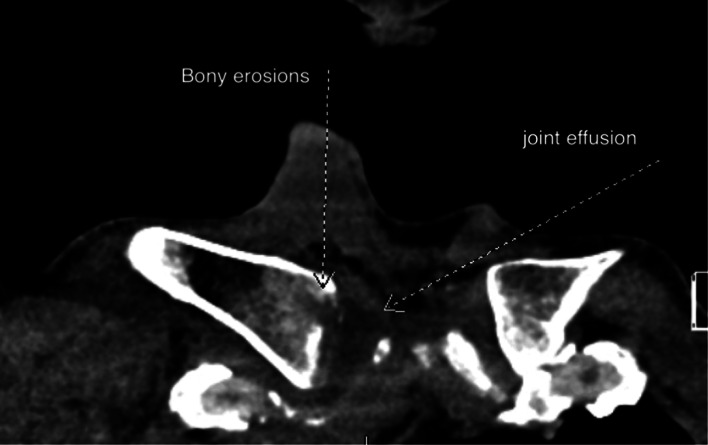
Computed tomography revealing joint effusion, bony erosions in the clavicle and adjacent sternal manubrium.
